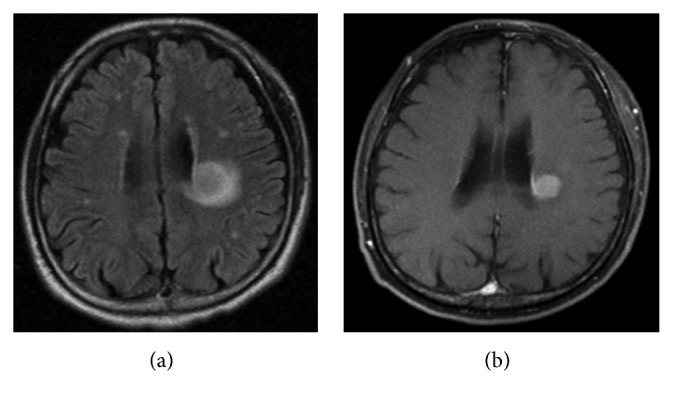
Magnetic resonance imaging (MRI) for CNS relapse. (a) Fluid-attenuated inversion recovery image showing a 2.3-cm mass in the infarction lesion in the white matter near the left lateral ventricle. (b) Contrast-enhanced MRI showing an evenly enhanced mass.
